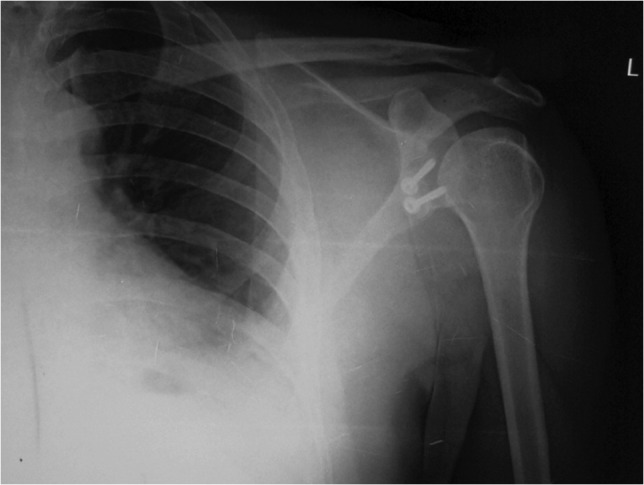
Post-operative radiograph with graft and screws in place.
